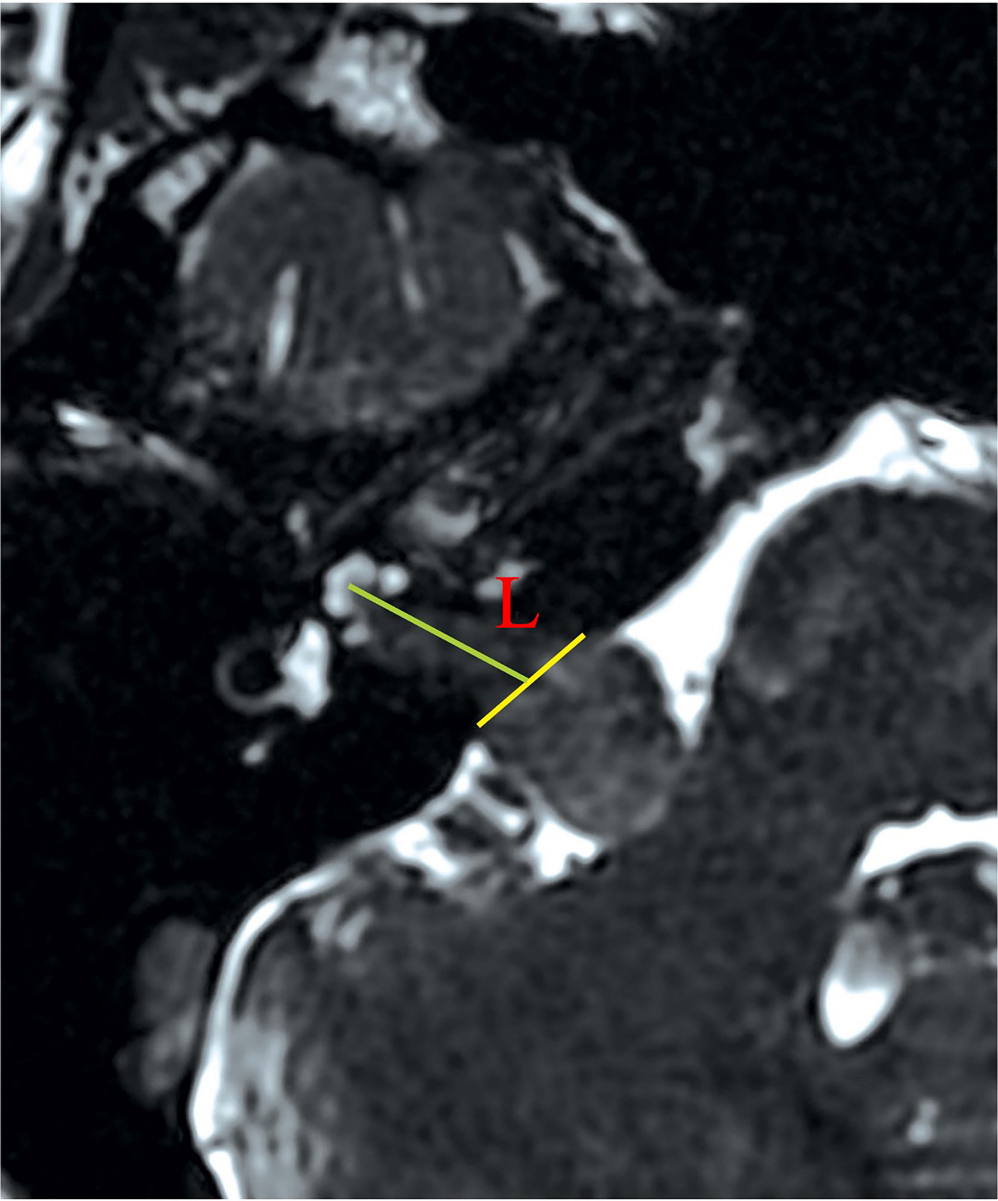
Measurement of the absolute tumor extension into IAC. On FIESTA-C, the slice with the most lateral extension of tumor is selected for measurement. An auxiliary line (yellow) is drawn between the anterior and posterior lip of the porus acusticus. The length of absolute tumor extension (labeled as L) is defined as the distance between the midpoint of the yellow line and the most lateral point of tumor extension. Defining the absolute extension of tumor in this way is different from assuming the extension of tumor as its projection on the posterior wall of IAC. The latter method appears ideal to pre-operatively define the length of the drilled posterior wall of IAC. However, the presence of tumor may erode the bony structure of the posterior wall of IAC, making delineation of the projection line difficult on pre-operative imaging studies. FIESTA-C, heavily T2-weighted fast imaging employing steady-state acquisition with cycle phase.
